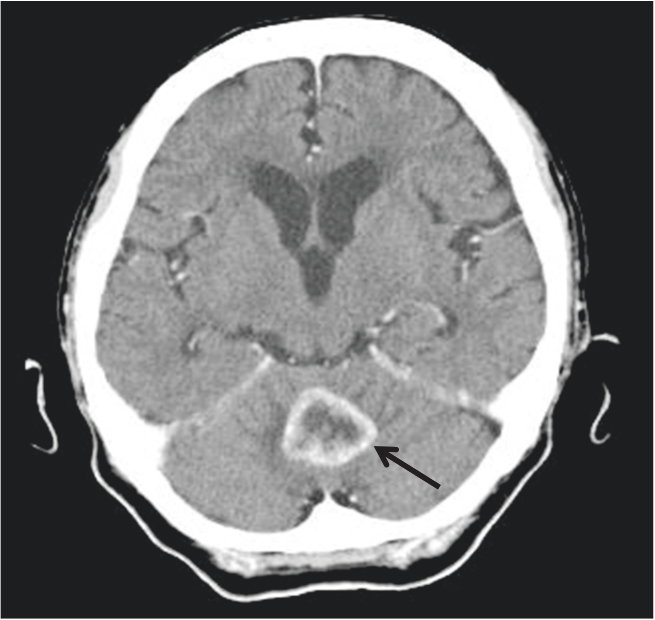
Computed tomography (CT) of the brain shows a tumor (diameter, 2.5 cm) with ring enhancement in the cerebellum (arrow).
